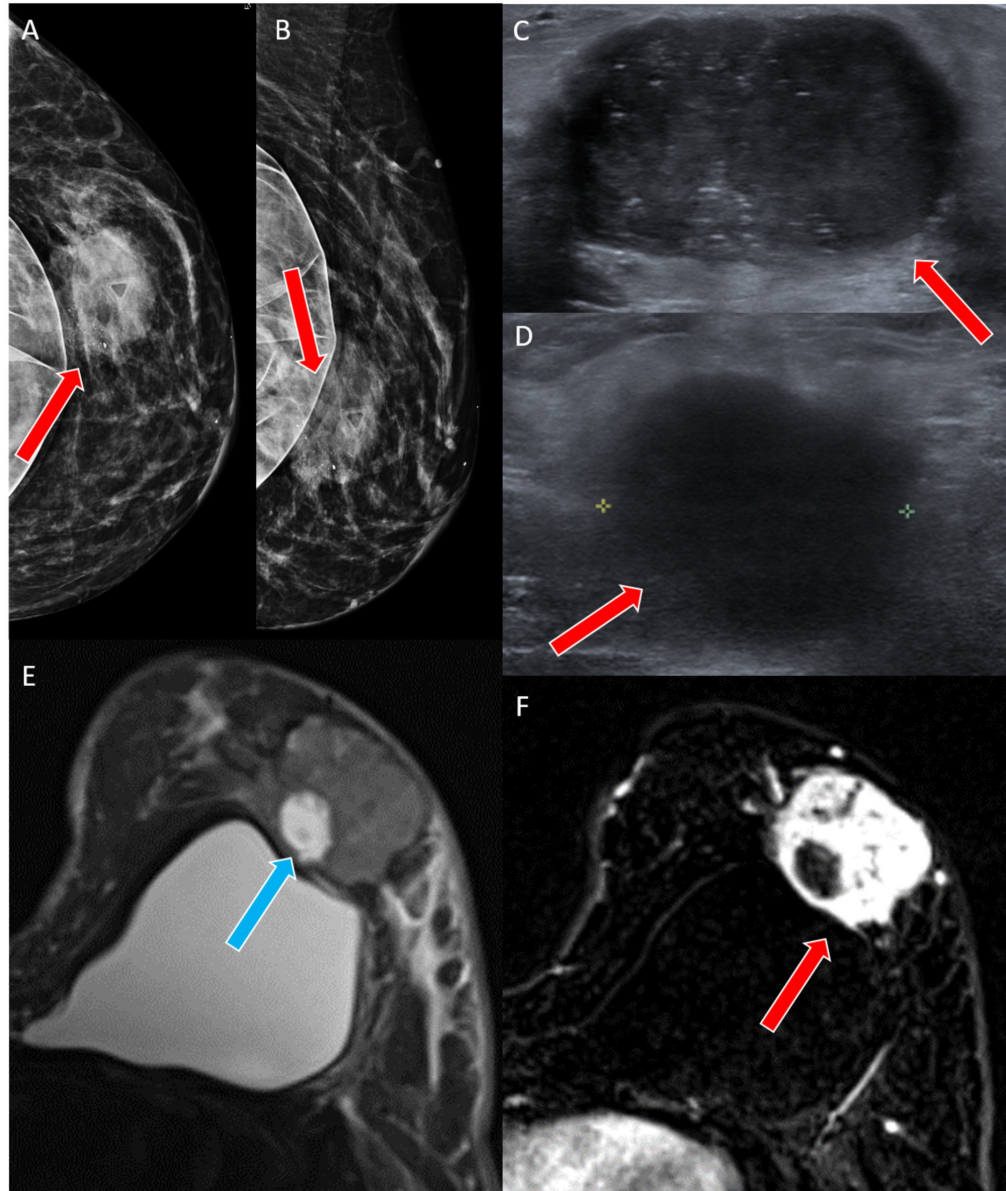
Case 2 imaging findings A, B: Craniocaudal and mediolateral oblique tomosynthesis views of the left breast show a round mass with partially obscured margins and pleomorphic calcifications (red arrows) in the central left breast corresponding to the area of palpable concern. The marker clip is from a reportedly prior benign biopsy. C: Ultrasound shows an oval, circumscribed hypoechoic mass (red arrow) with echogenic calcifications. D: A pathologic hypoechoic lymph node (red arrow) is seen in the left axilla on ultrasound. E, F: MRI shows a heterogeneously enhancing mass (red arrows) with an area of T2 hyperintensity (blue arrow) consistent with necrosis.
